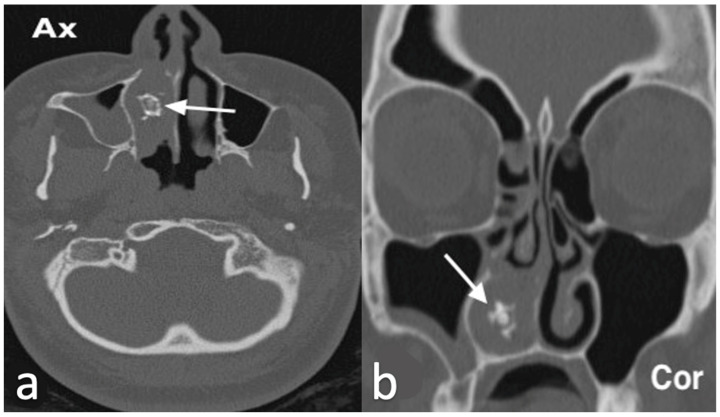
Rinolith in a male patient with history of right nasal obstruction, worsening with fetid purulent, both front and rear, rhinorrhea for about 2 years. On axial and coronal CT (a,b), the right nasal cavity is obstructed by an irregular calcified, hyperdense lesion (arrow) with mucosal thickening and, due to the mass effect, the medial wall of the right maxillary sinus is slightly compressed and laterally bowed. Nasal endoscopy confirmed the presence of a huge rinolith with wooden consistency in the right nasal cavity adhering to the nasal septum.
